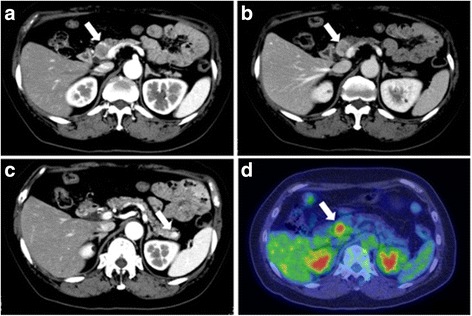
Radiological images of masses in the pancreas and the left adrenal gland. a-c Contrast-enhanced computed tomography of the pancreas. a Arterial phase shows a mass with peripheral enhancement (*arrow*) in the pancreatic head. b Portal phase demonstrates a well-defined mass with weak and heterogeneous enhancement (*arrow*) in the pancreatic head. c In the pancreatic tail, arterial phase reveals a rounded and well-delineated mass with marked enhancement (*arrow*). d Fluorine-18 fluorodeoxyglucose positron emission tomography imaging shows a positive mass (*arrow*) in the pancreatic head
